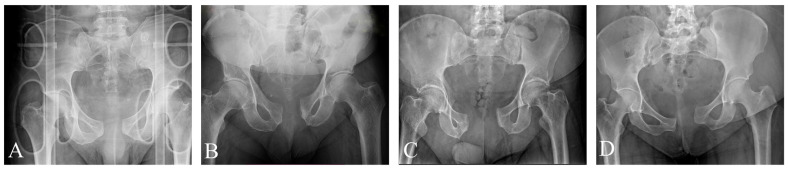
AP pelvic X-rays of patients upon admission. Patients 1 (A), 2 (B), and 4 (D) suffered unilateral injuries, coded as 61B2.3 according to the AO/OTA classification [10], while Patient 3 (C) suffered bilateral (61B3.3) injury, indicating bilateral involvement with posterior instability.
